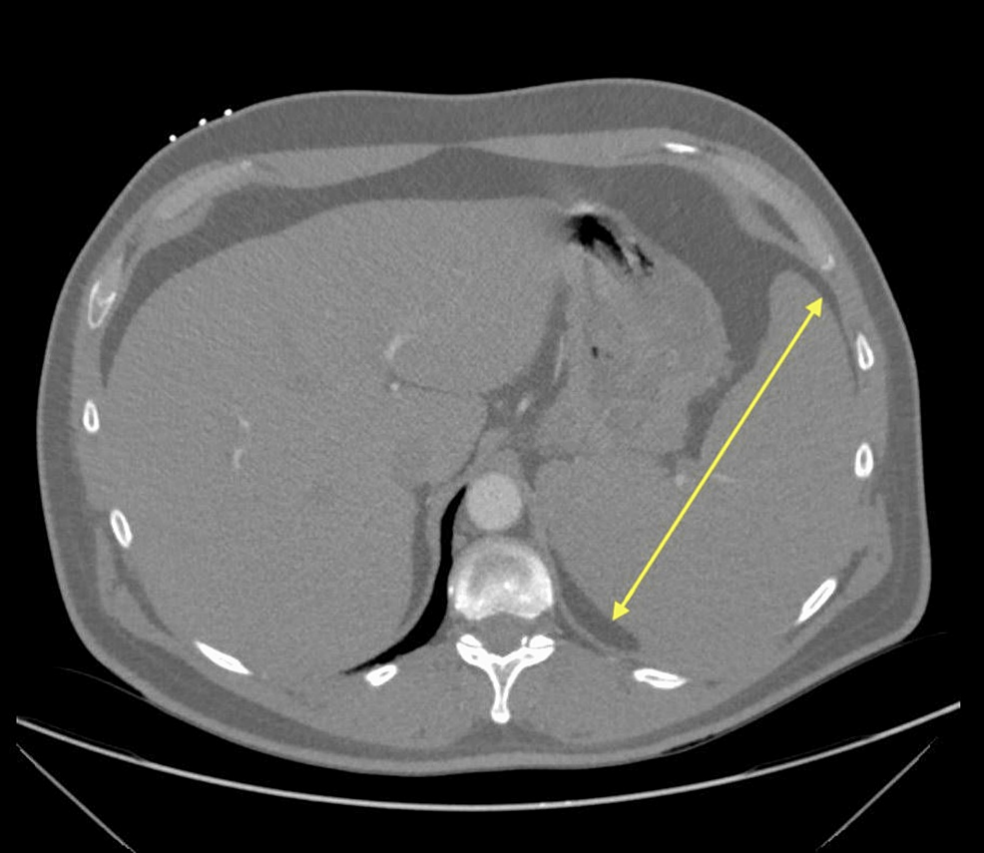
Initial CTA of chest showing splenomegaly, demonstrated with yellow line CTA: CT angiography
